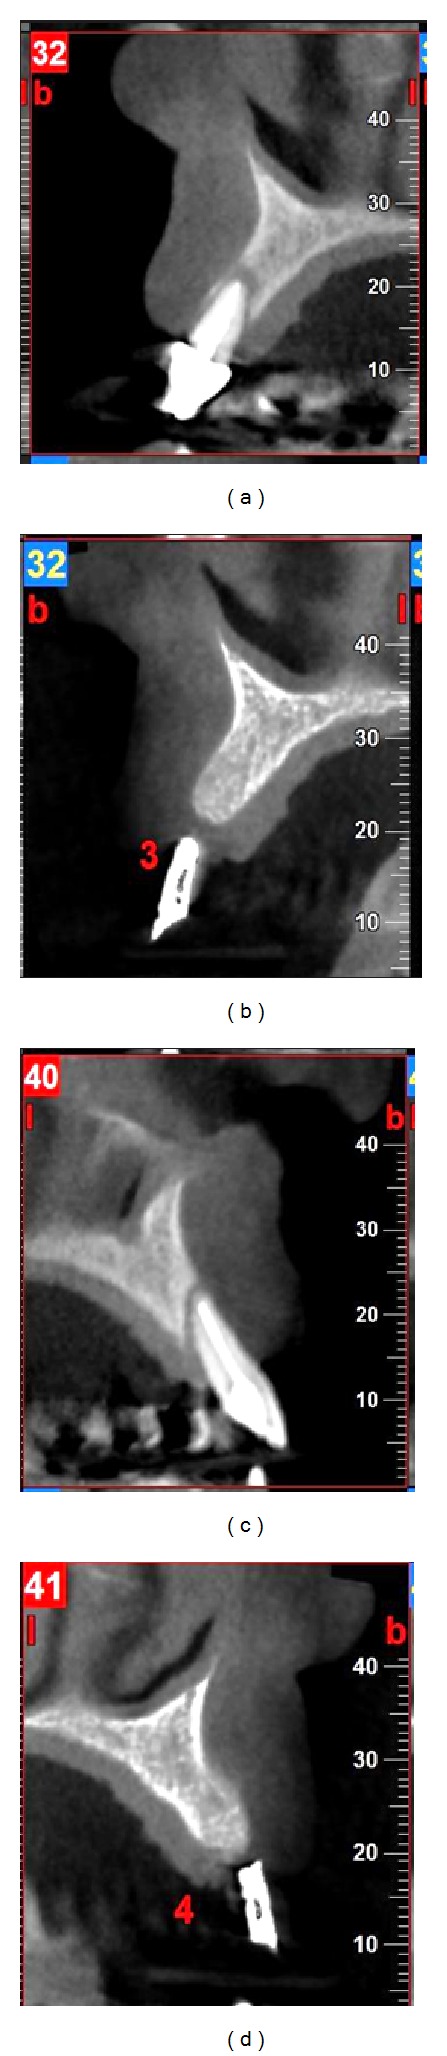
Radiographic views of Case  3. CBCT images at (a, c) pretreatment and (b, d) 6 months postoperatively.
